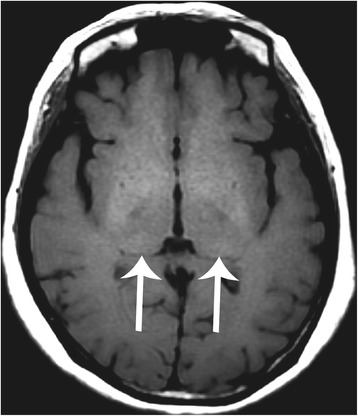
A middle-aged female patient with IVS4+919G>A-type Fabry disease with hypertrophic cardiomyopathy. Brain magnetic resonance axial T1-weighted image reveals the pulvinar sign with high signal changes at the bilateral posterior thalamus (arrows)
